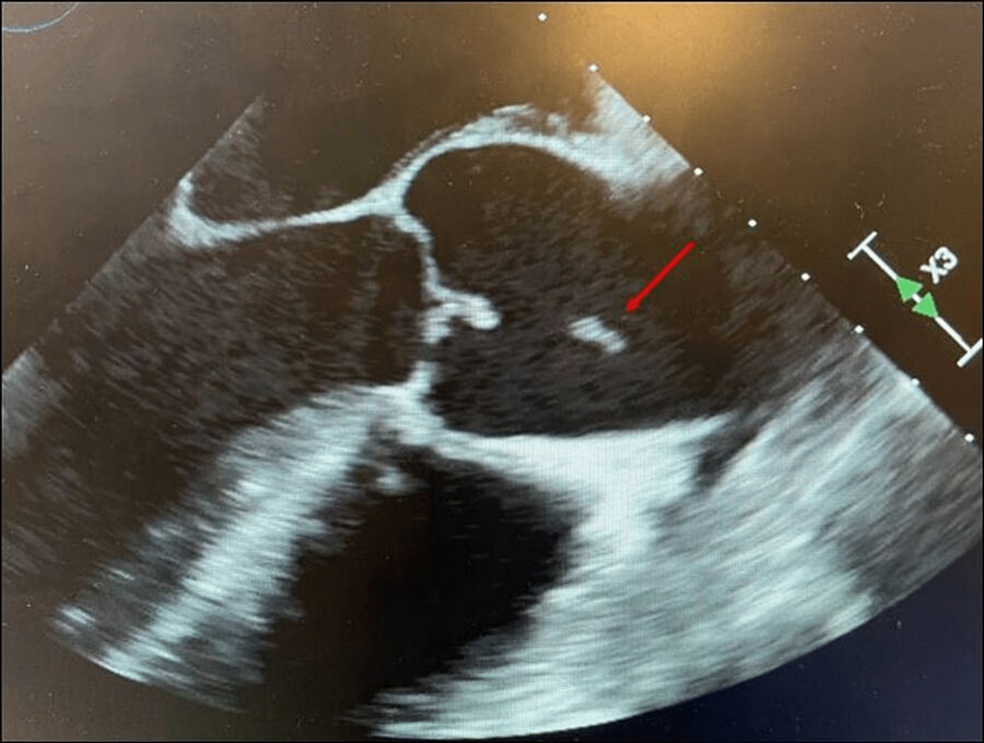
Transesophageal echocardiographic view showing the aortic valve with a mobile echo density (red arrow).
